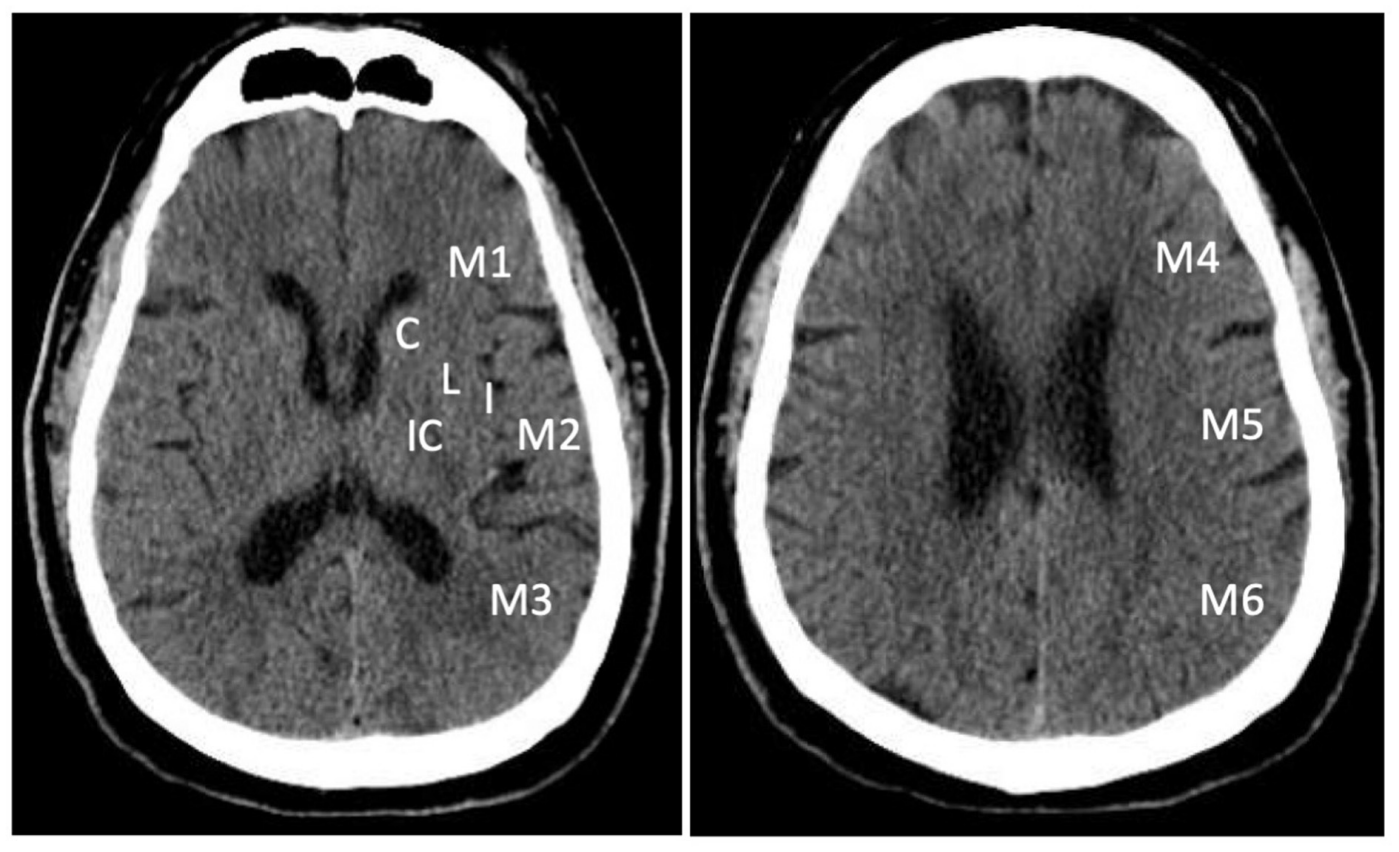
Alberta Stroke Program Early Computed Tomography Score (ASPECTS). The scoring system divides the MCA territory into 10 zones at ganglionic and supra-ganglionic levels: 6 for cortical regions (M1- M6), and 4 subcortical regions (C, caudate; L, lentiform; IC, internal capsule; and I, insular ribbon).
